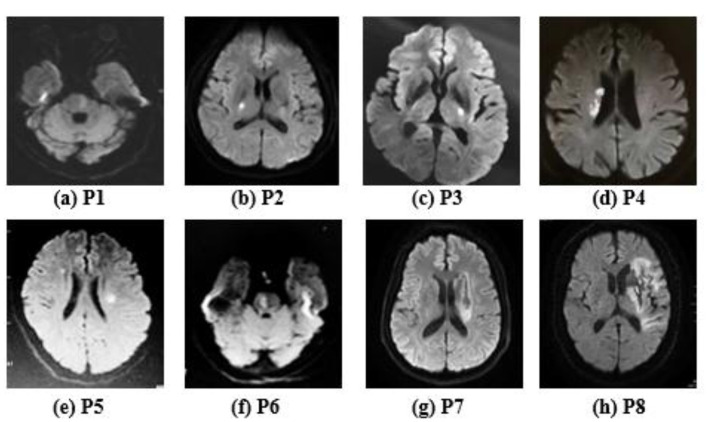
Cranial MRI images of eight stroke patients with hemiplegia. (a) P1. (b) P2. (c) P3. (d) P4. (e) P5. (f) P6. (g) P7. (h) P8.
